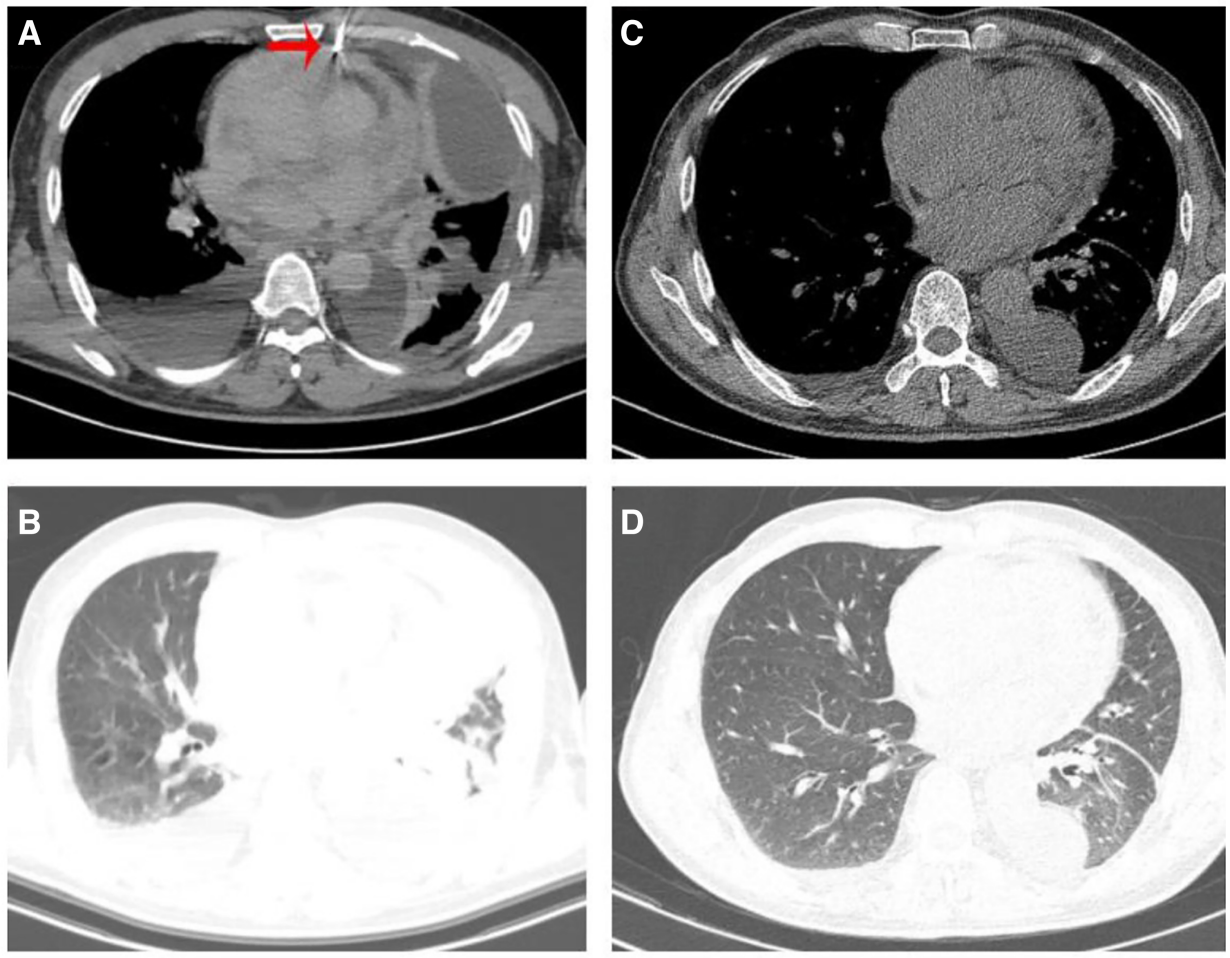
(A) Patient underwent CT guided pericardial biopsy. (A,B) Chest CT scan revealed bilateral pleural effusion, pericardial thickening, and pericardial effusion before treatment. (C,D) Chest CT showed a significance reduction in pleural and pericardial effusion after receiving one cycle of anlotinib treatment.
